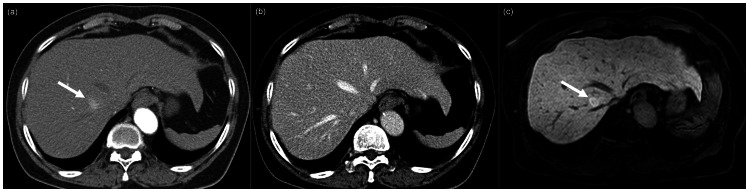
73-year-old female with incidental liver mass. (a) Arial phase CT image shows ill-defined hypervascular lesion in segment 8 of the liver (arrow). (b) The lesion shows isoattenuation on portal venous phase and both reviewers diagnosed this lesion as arterioportal shunt on CT with a confidence level of 4 and 3, respectively. (c) However, this lesion presented with high signal intensity compared to the adjacent normal liver on hepatobiliary phase of MR image (arrow). The diagnosis was changed to FNH on MR with a high confidence level of 5 by both reviewers.
